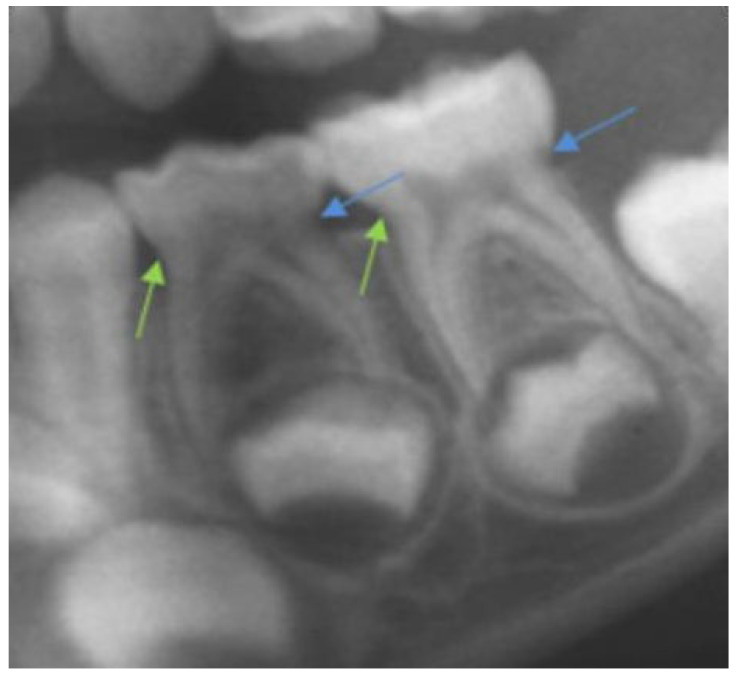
Magnification detail of an orthopantomograph, in which the reference of the dentin–enamel junction is observed in mesial (green arrows) and distal (blue arrows).
